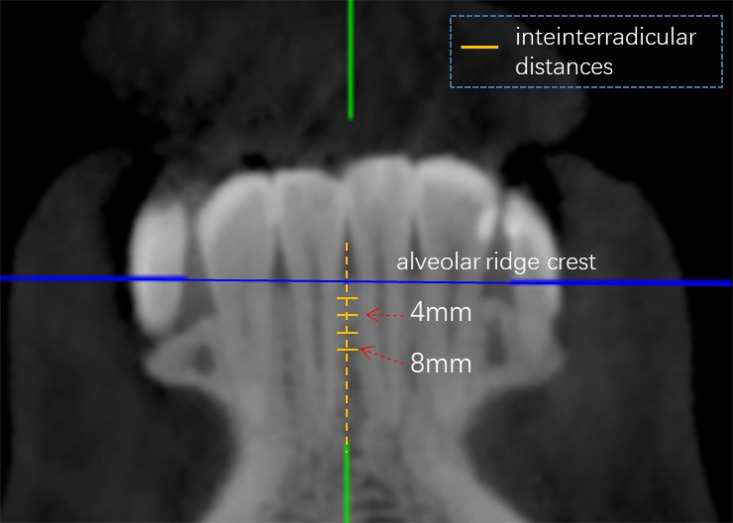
CBCT measurement: interradicular distance in coronal view.
